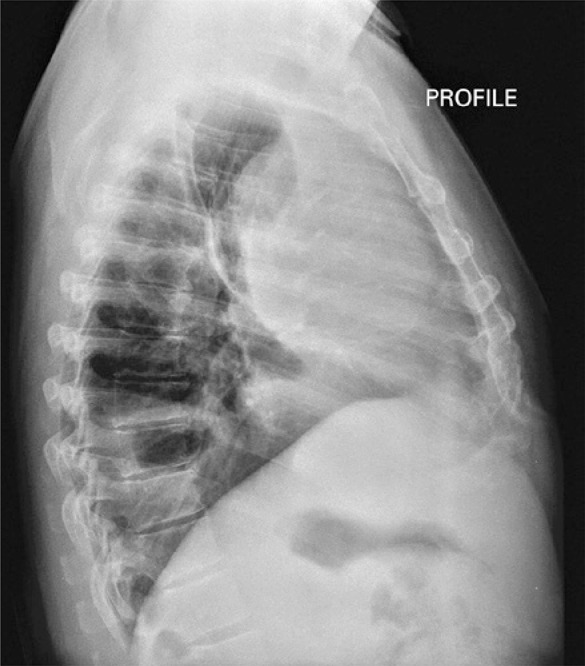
Profile of the thorax radiography showing homogenous densification located in anterior mediastium suggesting round contouring large mass
